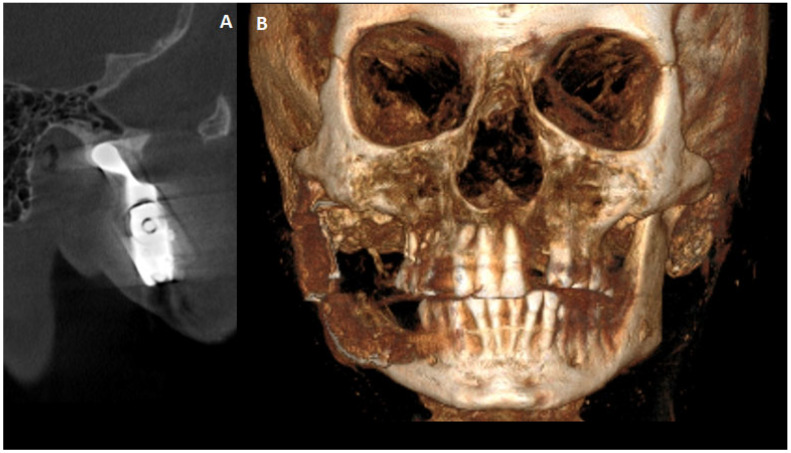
A final 12-month postoperative CBCT to evaluate the final results from surgery. (A)—CBCT sagittal scan with good TMJ position in the central spot of the glenoid fossa. (B)—CBCT-3D reconstruction with good bite, proper anterior facial height, and stable occlusion. In some cases, procedures concerning total temporomandibular joint replacement surgery (TMJR) greatly influence patients’ quality of life (QOL) [7]. Those aspects are related to improved mouth opening, the ability to bite and chew, restored balanced occlusion, and improved oral food intake. Since the concept of standard-made TMJ endoprosthesis is less common than it has been previously, it is worth knowing that in this case of Gorlin-Goltz syndrome, this approach is still worth considering. For example, if any OKC recurrence or new OKC cystic lesion near the TMJ prosthesis is found, it is always possible to change the device for a custom-made one or change the plating shape and length to keep the standard one in place. All TMJ alloplastic devices have many benefits that have been discussed widely in the literature.
